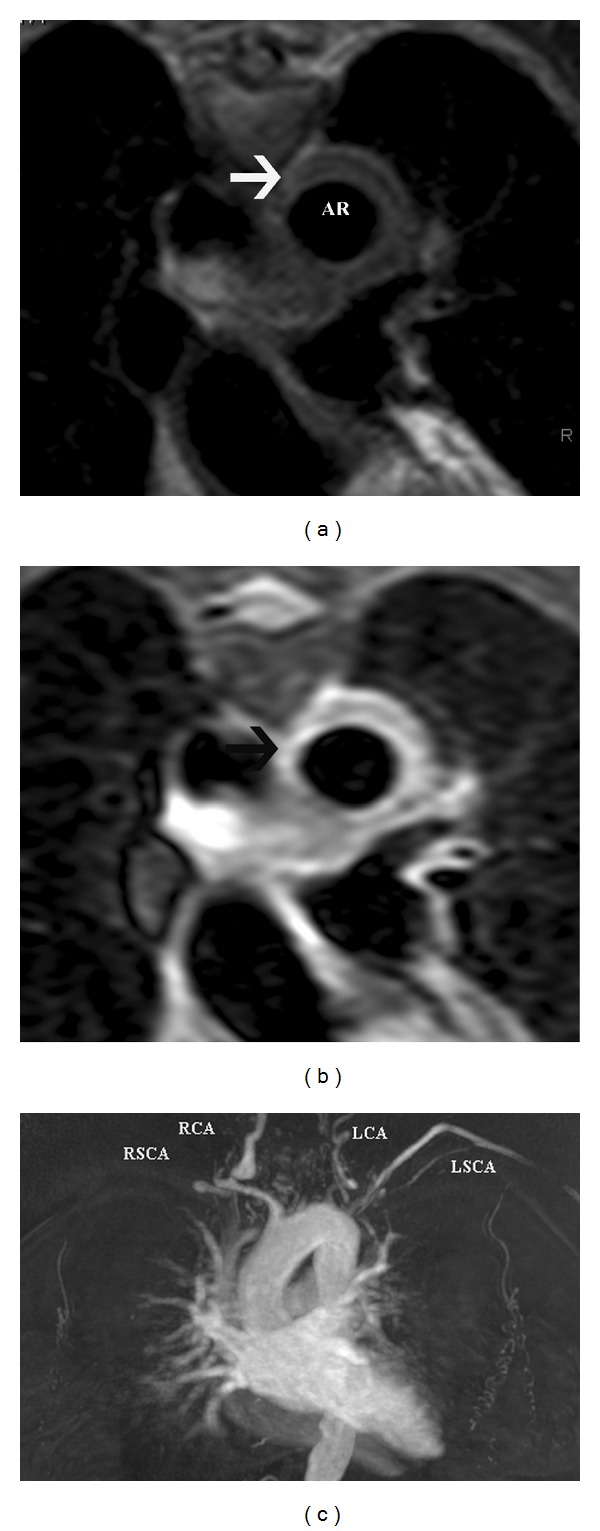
Takayasu   arteritis—(a) axial T2-weighted double inversion recovery black blood image in a 31-year-old female patient with Takayasu arteritis shows severe circumferential thickening of the aortic arch (arrows). (b) T2-weighted triple inversion recovery black blood image in the same patient with Takayasu arteritis shows very high signal of the thickened aortic arch (arrows), which is consistent with edema, indicative of active arteritis. (c) 3D contrast enhanced MR angiography images of the arch demonstrate severe stenosis of the left common carotid (LCA), right common carotid artery (RCA), and proximal left subclavian artery (LSCA) with occlusion of the right subclavian artery (RSCA).
